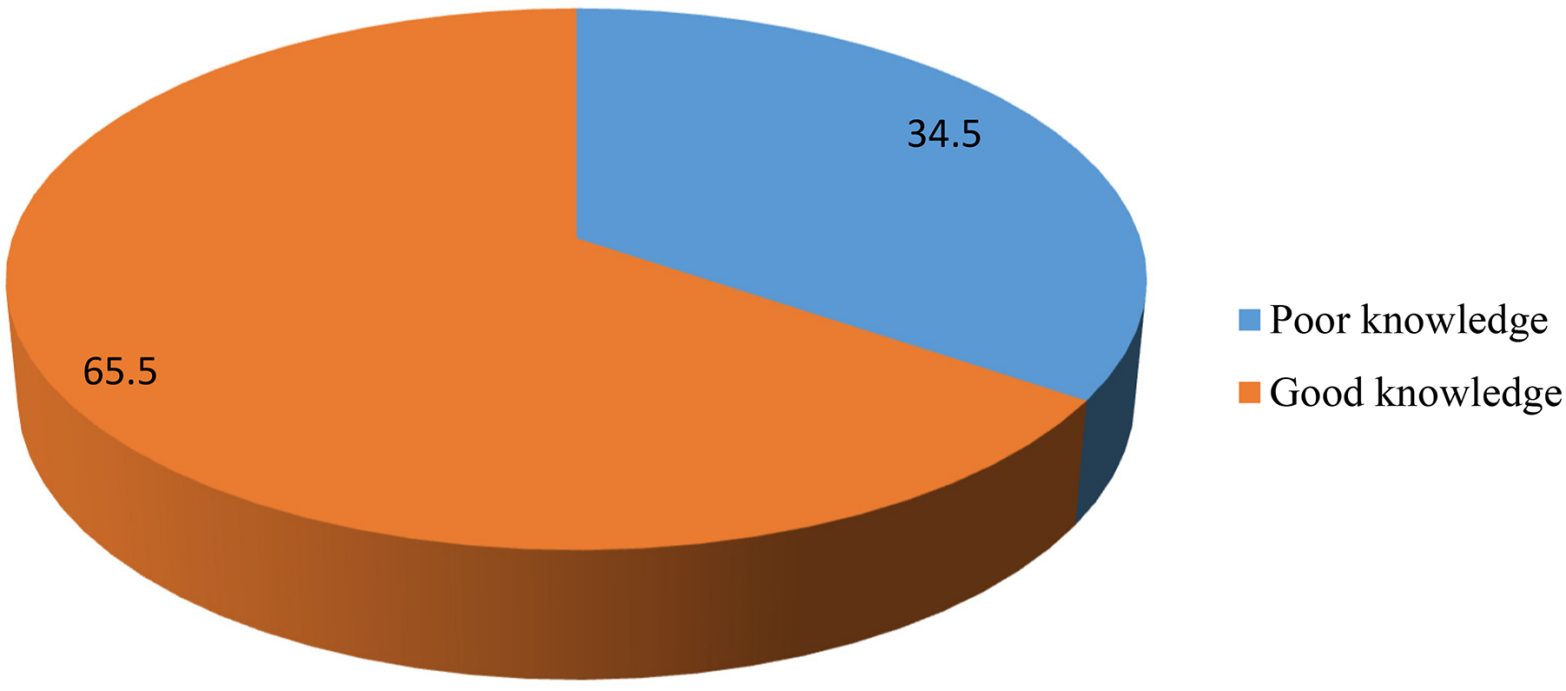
Knowledge of obstetric ultrasound finding among pregnant women attending ANC in selected South Wollo Zone Public Hospitals, 2023.
